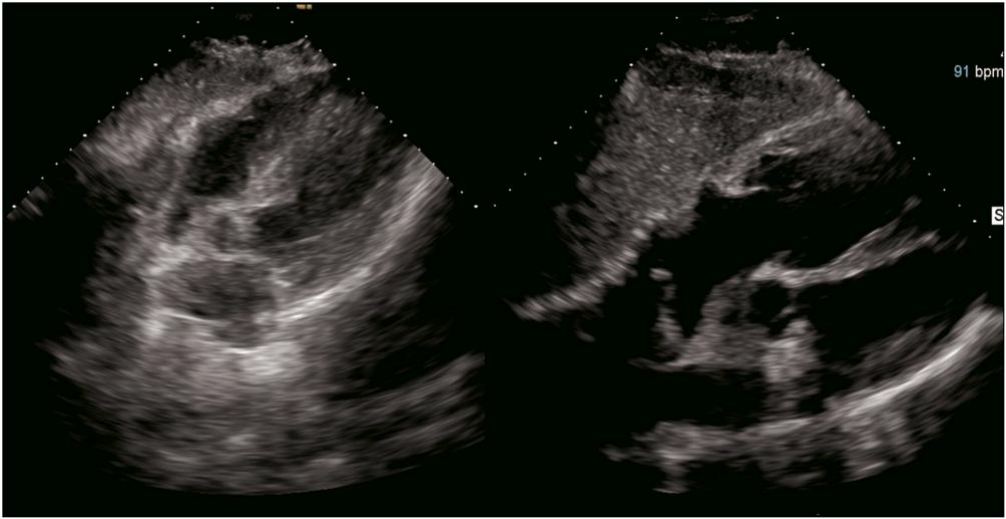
Echocardiograms (subcostal view) obtained before immunotherapy (left) and after admission (right) to demonstrate RV dilation.
